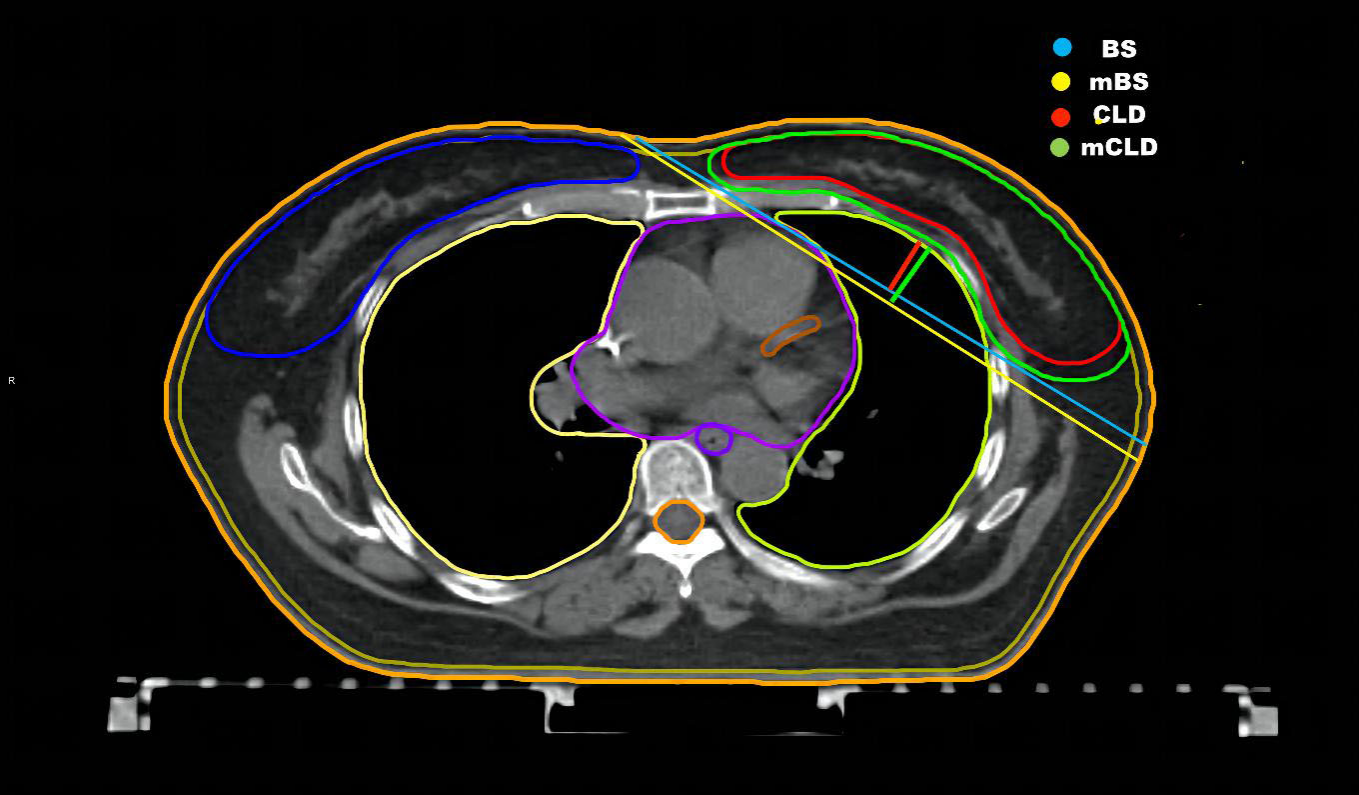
Anatomic parameters in the supine CT. The central plane from the low edge of clavicular head to the cardiac apex in the supine CT. The breast separation (BS) is the distance between entry points of two opposing beams on the central plane. The central lung distance (CLD) is the perpendicular distance from chest wall to the posterior boarder of the tangential fields.The modified breast separation (mBS) is the distance from the border of the sternum and the anterior border of latissimus dorsi then extending to skin.The modified central lung distance (mCLD) is the maximum perpendicular distance from BS to the posterior part of the anterior chest wall.
